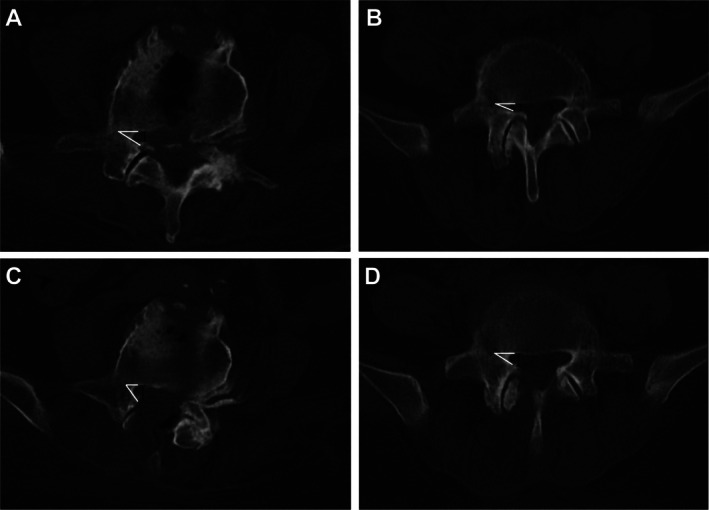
Preoperative and 1‐month postoperative axial CT at L4–5 showing lateral recess height and angle changes. (A, C) NON‐NAV, preoperative (A) and postoperative (C). (B, D) NAV, preoperative (B) and postoperative (D).
